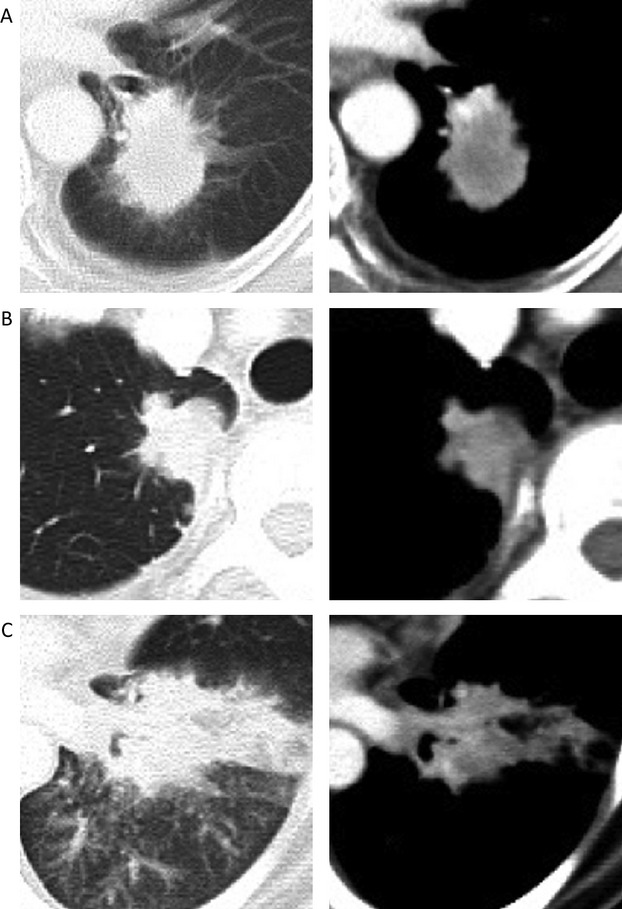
Thoracic CT findings from representative patients in *ALK*-positive NSCLC. (A) mass, (B) nodule, and (C) consolidation. ALK, anaplastic lymphoma kinase; NSCLC, non-small-cell lung cancer.
